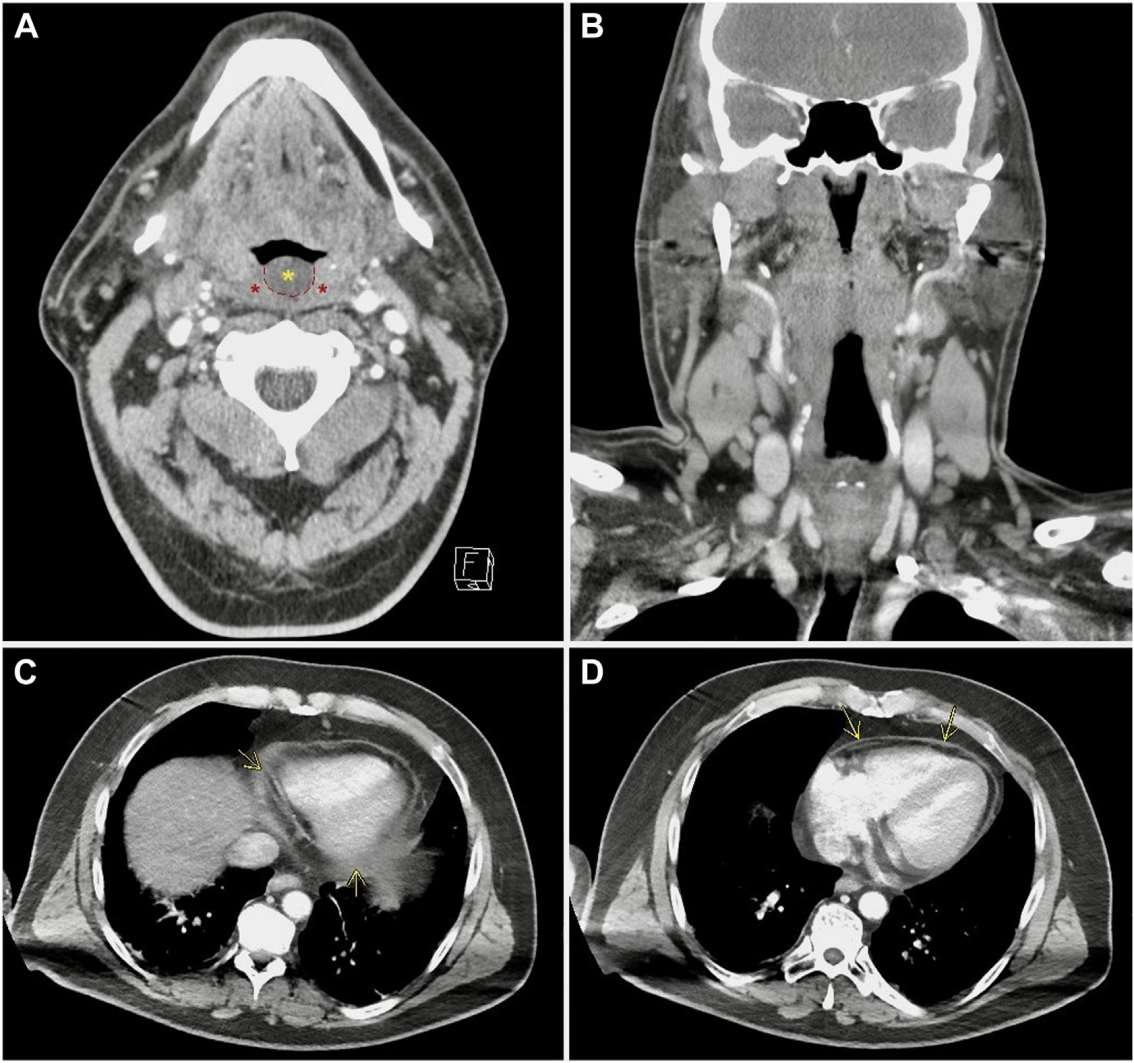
A: Computed tomography with intravenous contrast with a transverse view revealing bilateral thickened and edematous tonsils (red asterisks) and uvula (yellow asterisk). B: Coronal view revealing concomitant pronounced reactive cervical lymphadenopathy expected in Epstein-Barr virus infection. C, D: Transverse views of the mediastinum revealing new trace pericardial effusion with enhancement and thickening of the pericardial sac (*yellow arrows*).
